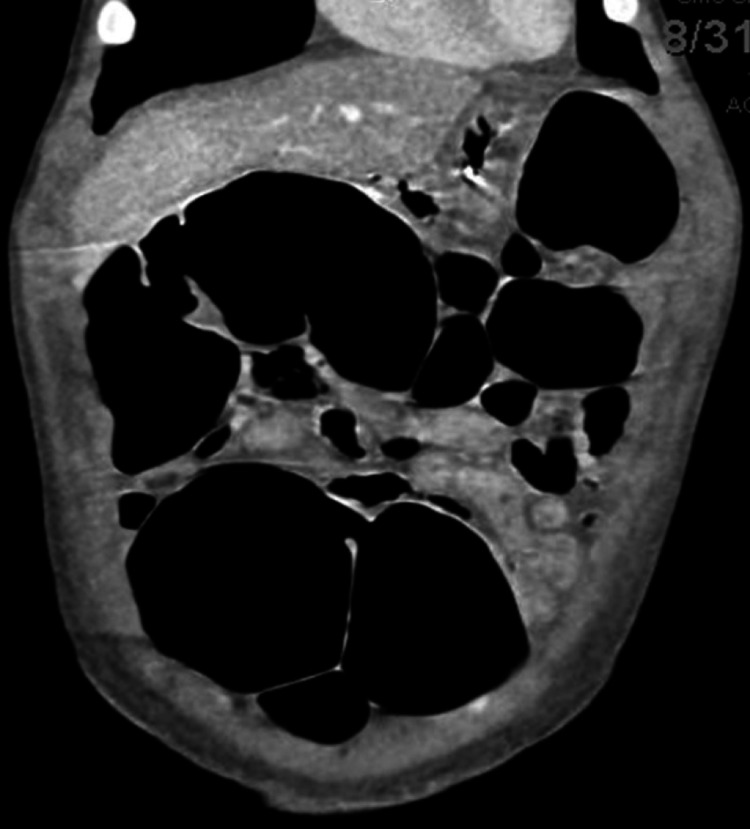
Abdominal sagittal CT scan image showing colonic distention CT: computed tomography
